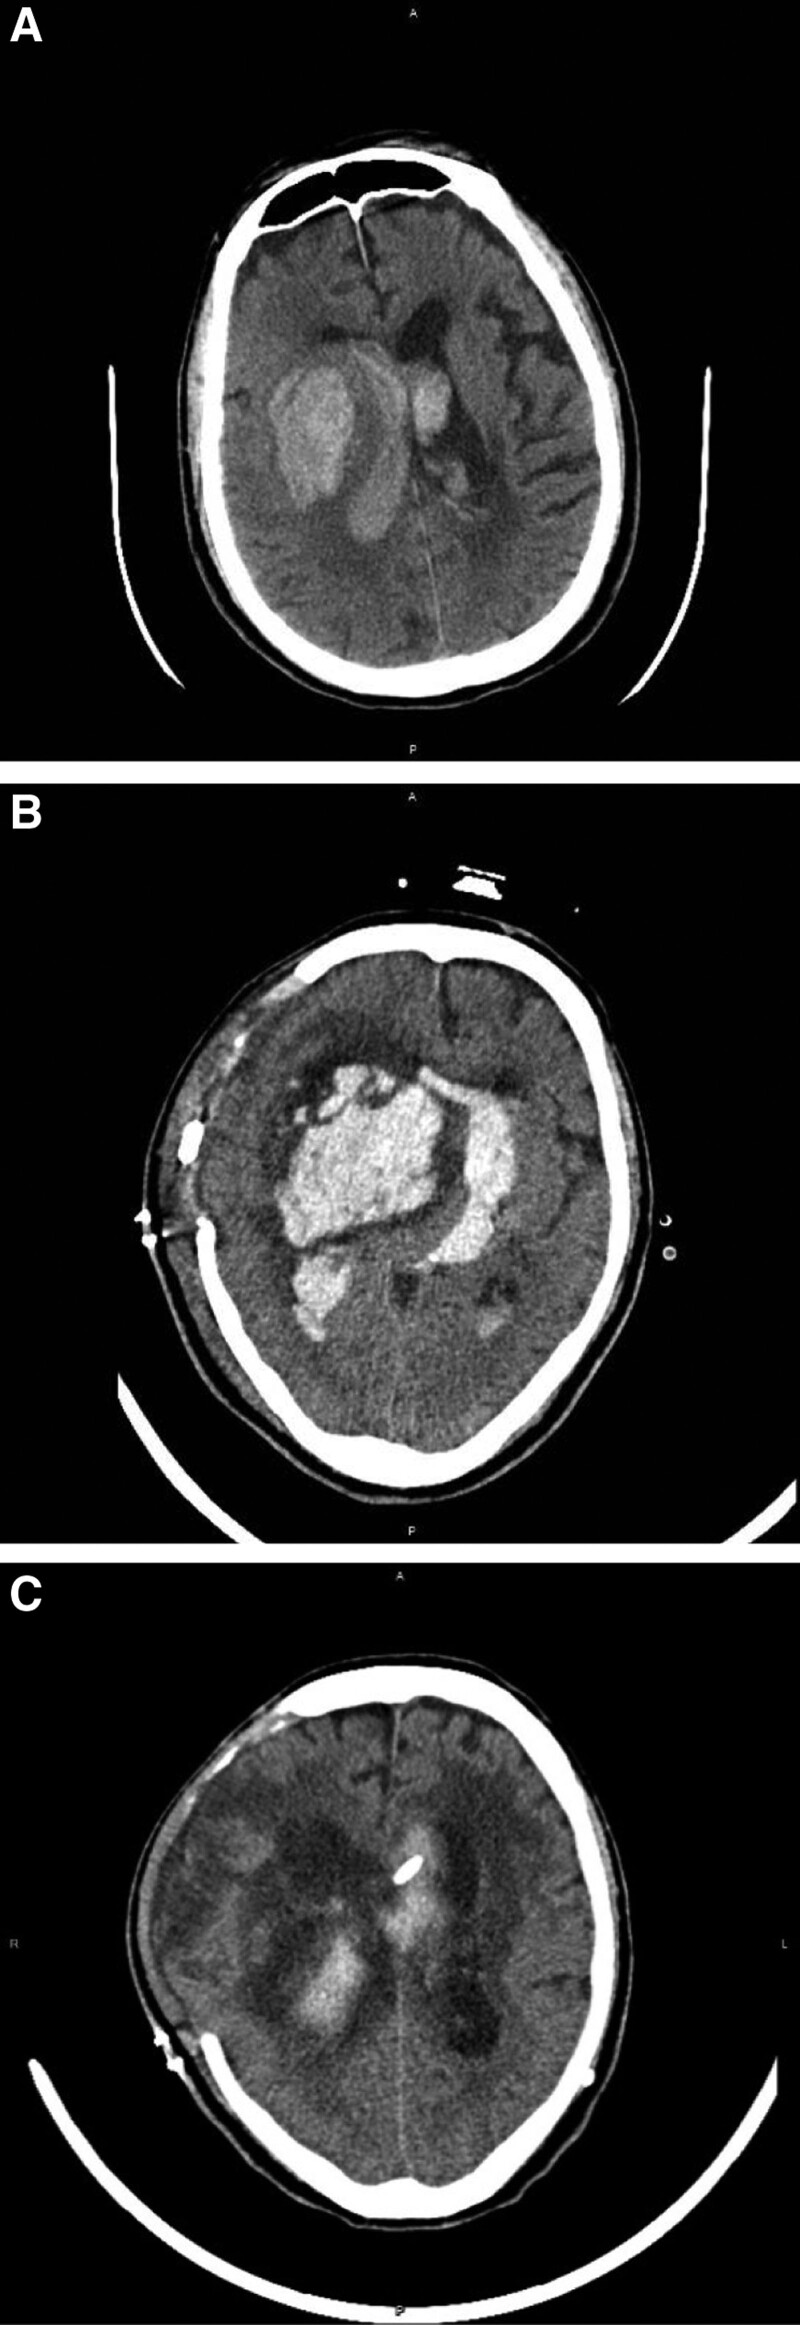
(A) The 22nd patient in our study, is a 73-year-old male with GCS of E2M4V1 when he was arrived to hospital, suffered from spontaneous right putamen ICH. (B) He received decompressive craniectomy and EVD, and the timing of the operation was about 122 min. However, he was still in a coma, and repeated brain CT data showed a rebleeding hematoma on day 3. (C) The patient was received reoperation of removal of hematoma and check bleeding. After the operation, his GCS showed improved (E1M2VT to E2M4VT). The patient suffered from worsening neurological symptoms after we clamp the EVD tube. The following brain CT revealed persistent hydrocephalus with dilated lateral ventricle; he then received the VP shunting operation. CT = computerized tomography, EVD = external ventricular drainage, ICH = intracranial hemorrhage, GCS = Glasgow Coma Scale, VP shunting = ventriculoperitoneal shunting.
